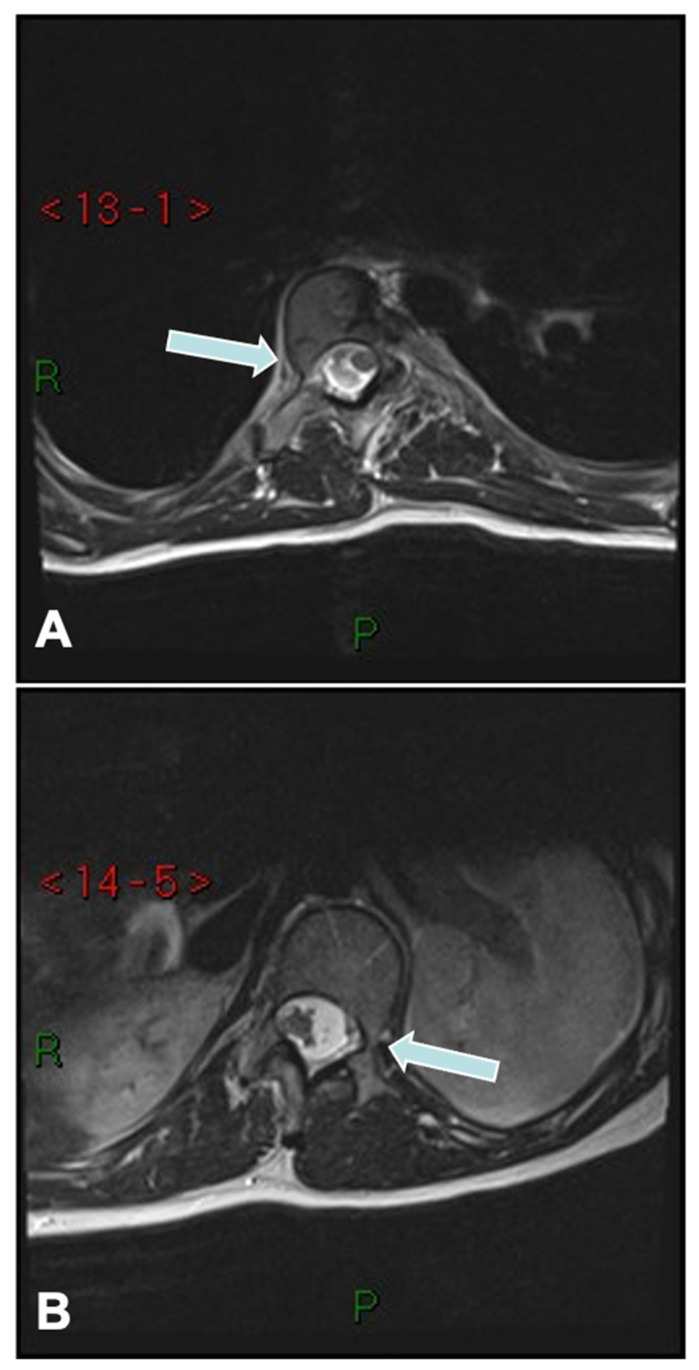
MRI cut sections at (A) D8 and (B) L1 showing relatively safer convex side pedicles (arrows) with the spinal cord lying at a distance from the convex pedicle when compared to its concave counterpart.
